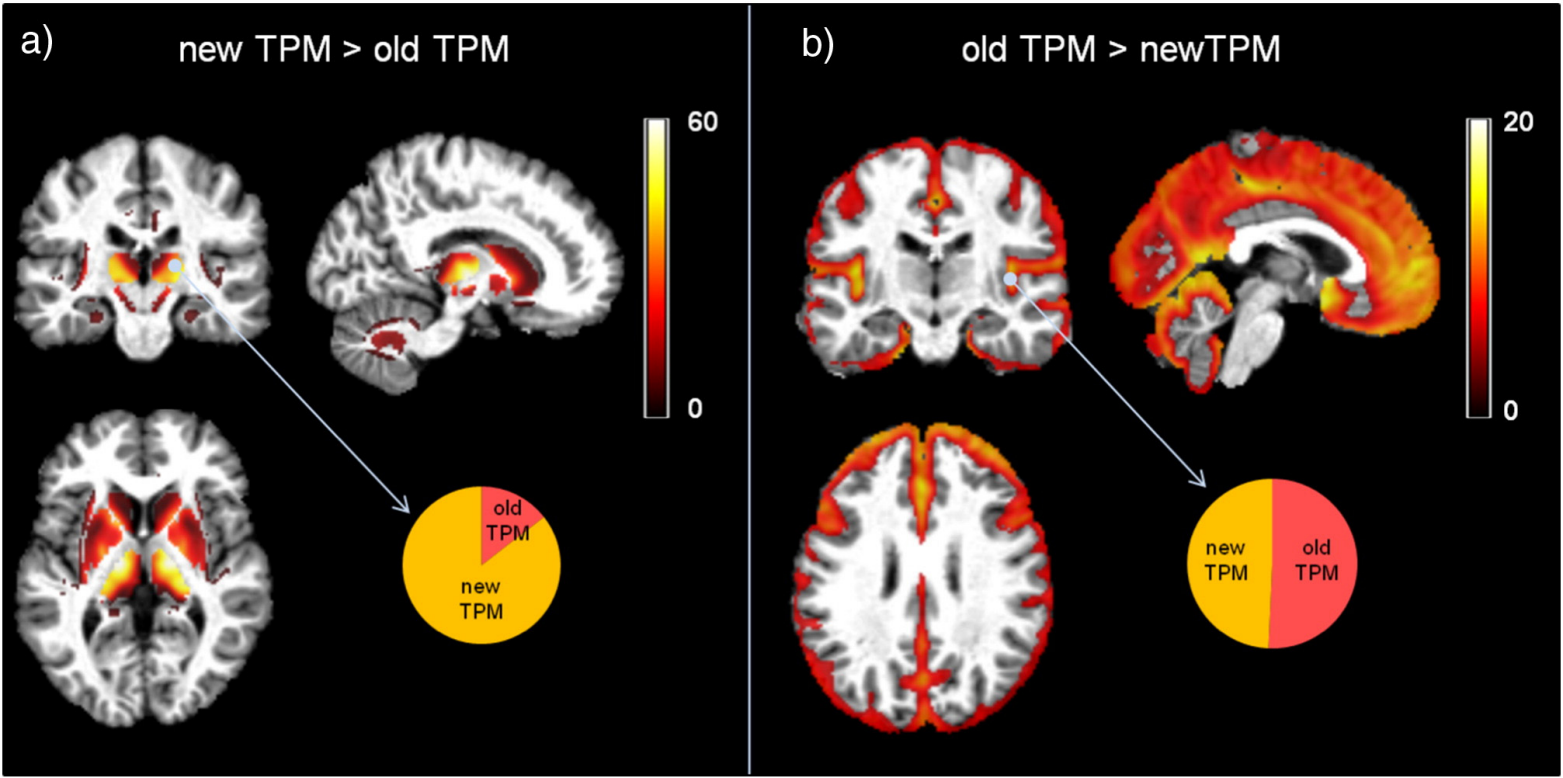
Statistical parametric maps of paired *t*-test between gray matter (GM) volumes estimated from T1-weighted data with new and conventional tissue probability maps (TPM) at statistical threshold of *p*_FWE_ < 0.05. (a) Increase of GM volume estimation, based on the new TPM, compared to the estimation based on conventional TPM. (b) Increase of GM volume estimation, based on the old TPM, compared to the estimation based on conventional TPM. The pie charts represent the effect size for the indicated brain location. All results are presented at *p*_FWE_ < 0.05.
